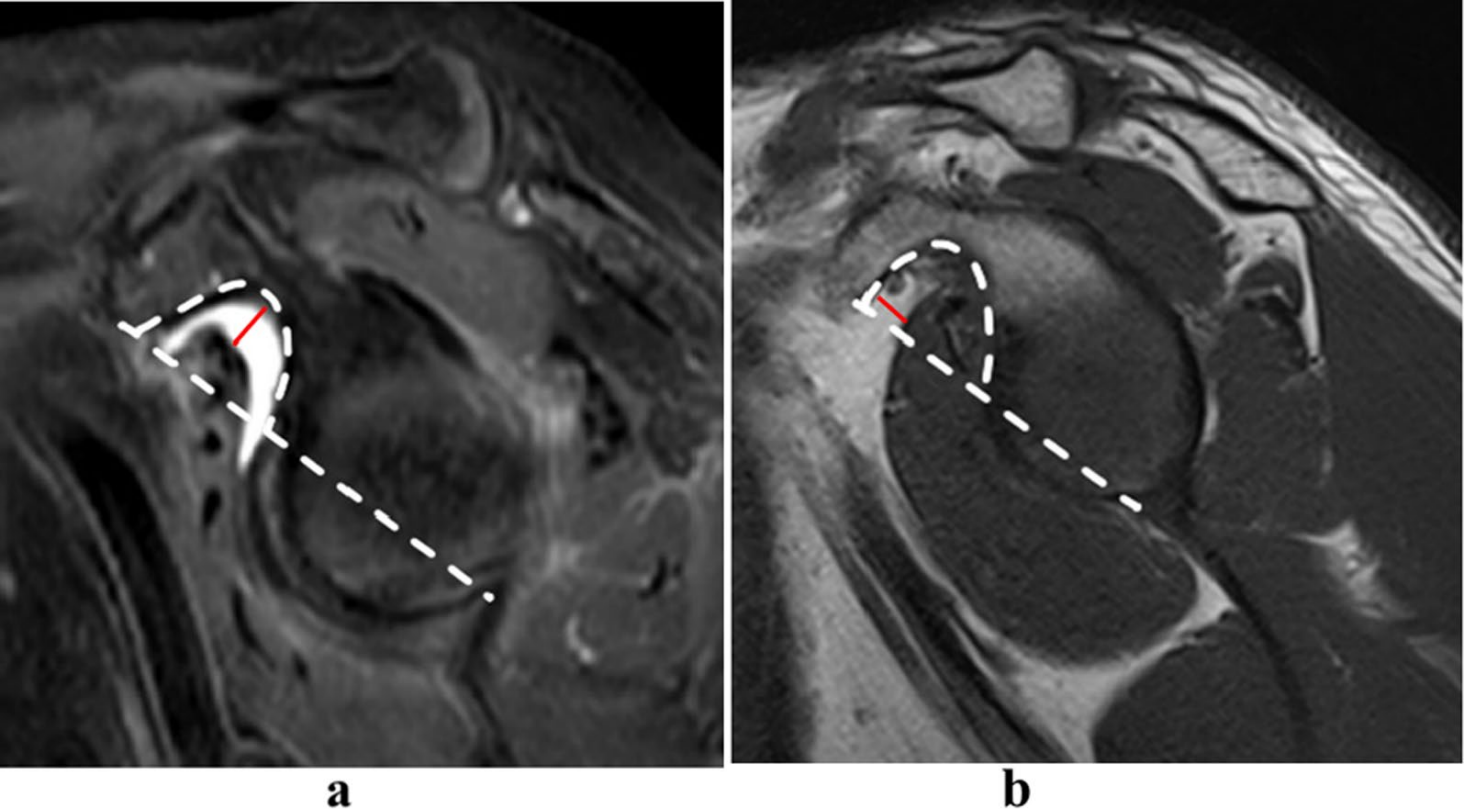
Quantitative measurements of fluid collection and fat accumulation on IM-WI/fs (a) and T1-WI (b) oblique sagittal MRI at the “coraco-glenoid arch” (CGA) slice. Both the coracoid process and glenoid are well seen and connected by the base of coracoid process on this slice. An arch line can be drawn along the inferior margin of the coracoid and glenoid, another reference line is made connecting the anterior inferior coracoid tip and the leading edge of the insertion of the triceps tendon, and the maximum distance of the fluid or fat accumulated in the coraco-glenoid arch is separately measured
